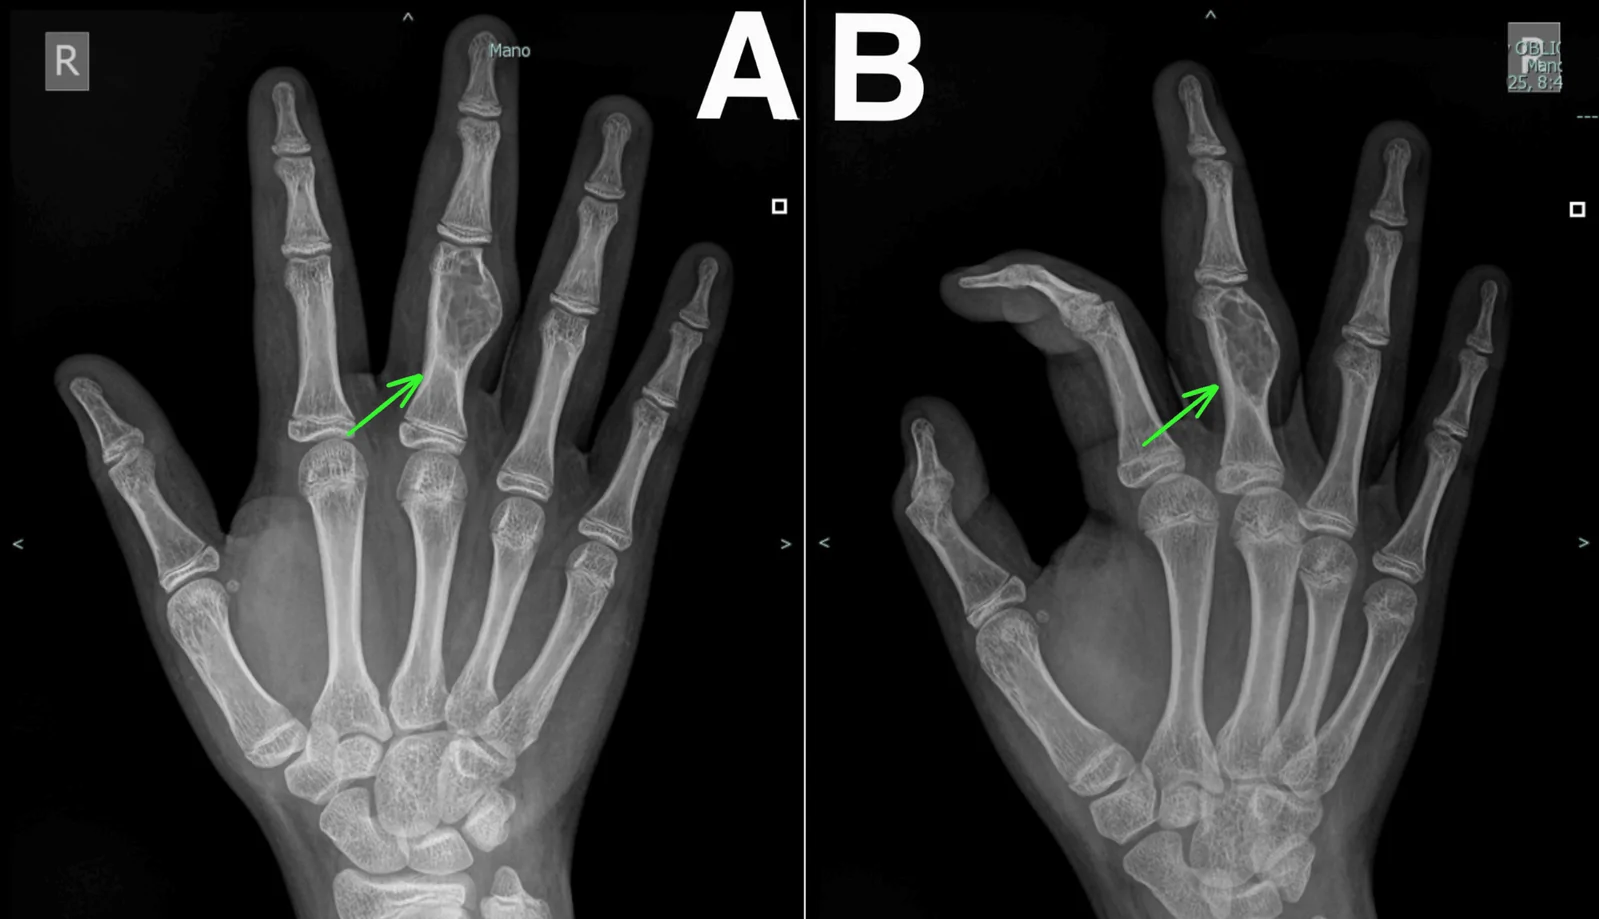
Preoperative radiographs. (A) Anteroposterior view. (B) Oblique view. Expansile lytic lesion in the proximal phalanx of the middle finger with expansion of the ulnar cortex and internal chondroid calcifications.
